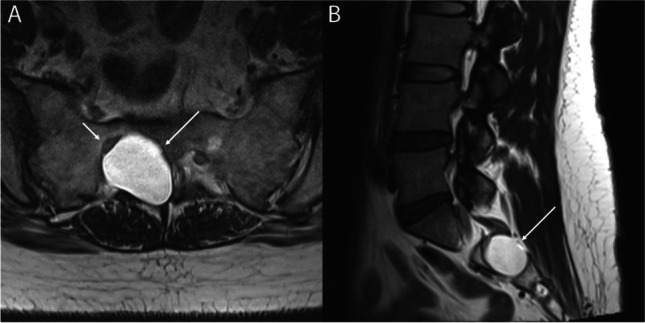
T2-weighted images of a Tarlov cyst. A Axial images shows the cyst expanding and remodeling the right S2 foramen and sacral canal (arrows) with the S2 nerve displaced and flattened (short arrow). Sagittal image (B) shows the relation of the cyst to the S2 foramen
